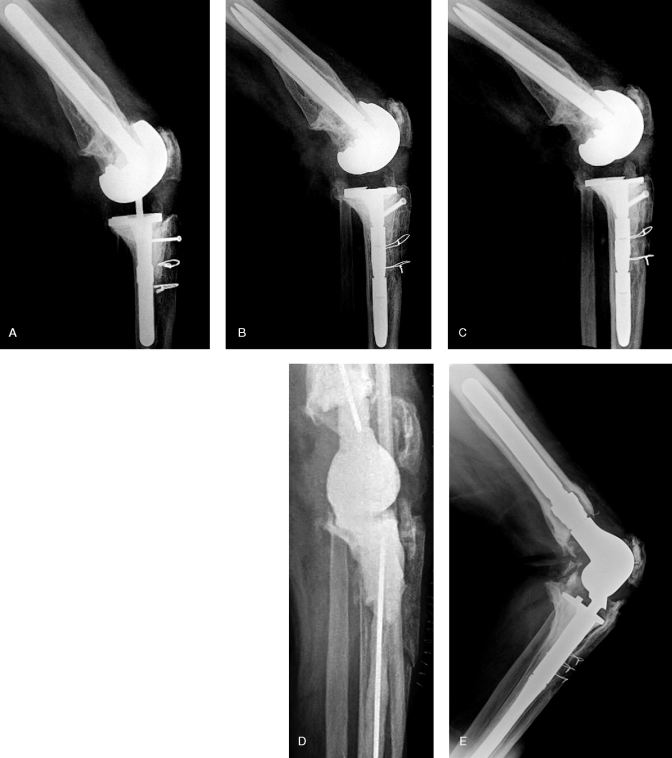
3 TTOs on the same knee. A. Healed primary TTO in a 57-year-old man who underwent RTKA of the left knee. A bicortical screw proximally and 2 wires distally were used for osteotomy fixation. B. 4 years later, implant loosening and instability developed, and another RTKA with a second TTO was performed. Fixation of the osteotomy was achieved with the same osteosynthesis method as the previous TTO. C. Complete consolidation of the osteotomized fragment was seen radiographically 6 months postoperatively. D. 2 years later, knee infection occurred. The implants were removed and an articulated antibiotic-impregnated cement spacer was inserted. A third TTO was performed during removal of the infected TKA and antibiotic cement spacer implantation. The osteotomy was left unfixed to avoid introduction of metallic fixation into a contaminated wound. E. Fixation of the osteotomy with 3 wires was performed during the second stage of RTKA. At the final follow-up 2 years postoperatively, the tibial tubercle was well healed.
